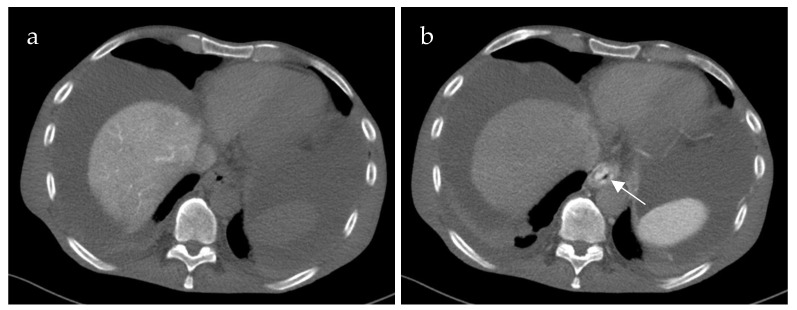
Computed tomography mesenterico-portography (a) and computed tomography splenoportography (b) of a 58-year-old patient with portal hypertension, portal vein thrombosis and portal vein confluence thrombosis. Esophageal varices (EV) are detected after contrast injection into the splenic artery ((b), white arrow). The EV do not show contrast enhancement after contrast injection into the superior mesenteric artery.
